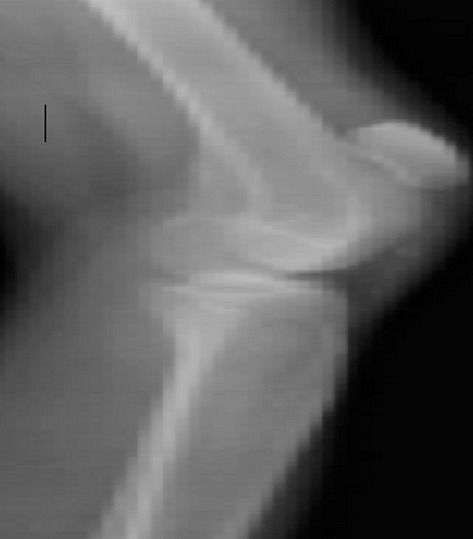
Radiographie de profil du genou droit objectivant la patella alta
